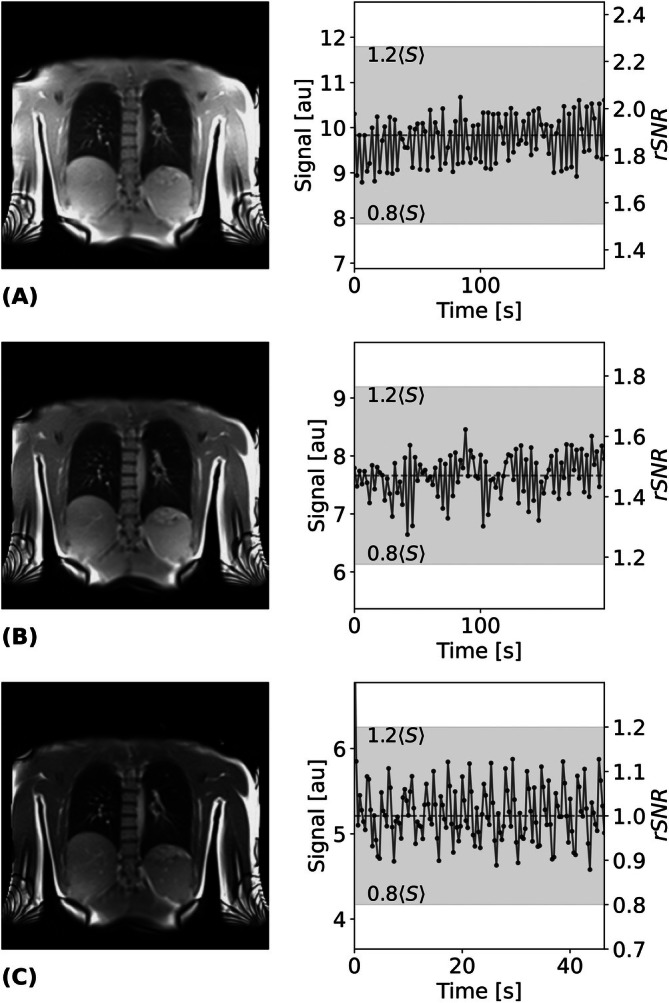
Example of CSC and MP MRI for one slice position of 10 mm slice thickness, acquired in free‐breathing. The images refer to the 100th image acquired in the timeline and the plots show the signal time course averaged over the segmented lung after image registration. The solid lines show the mean signal of all images used to calculate the rSNR for the CSC as compared to the time‐resolved scans. The same windowing was used for the images. (A) CSC with centric view ordering (100 images). (B) CSC with linear view ordering (100 images). (C) MP MRI with linear view ordering (140 images).
